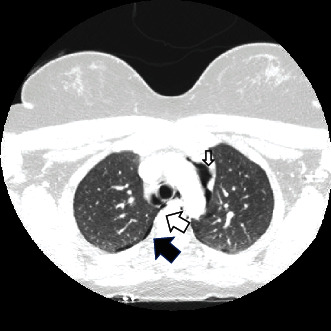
CT angiography of the head and neck. Small right apical pneumothorax (solid arrow) and pneumomediastinum (nonsolid arrows) are demonstrated.
